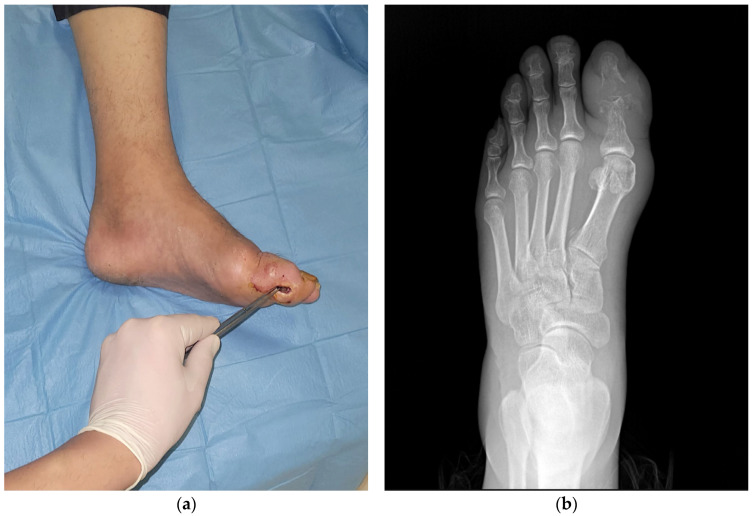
(a): Clinical image of a 68-year-old male patient with diabetic toe osteomyelitis. IDSA/IWGDF ulcer classification: Grade 3. The ulcer shows a positive probe-to-bone test. (b): Corresponding X-ray image of the same patient demonstrating findings consistent with osteomyelitis. Cortical irregularities, osteolytic lesions, and soft tissue edema are visible at the level of the interphalangeal joints.
